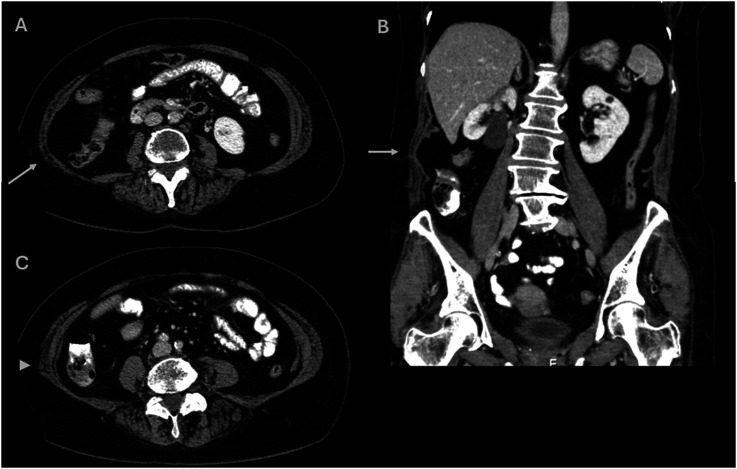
Example of bulge score 1 in axial and coronal reformatted CT images. A and (b) Moderate focal muscle atrophy (arrows) in the upper right abdominal wall, at L3 level in axial plane (a) and coronal plane (b). This was graded as bulge score 1 by both observers. (c) Axial plane at L5 level in the same patient shows only slight asymmetry (arrowhead) in muscle volume.
